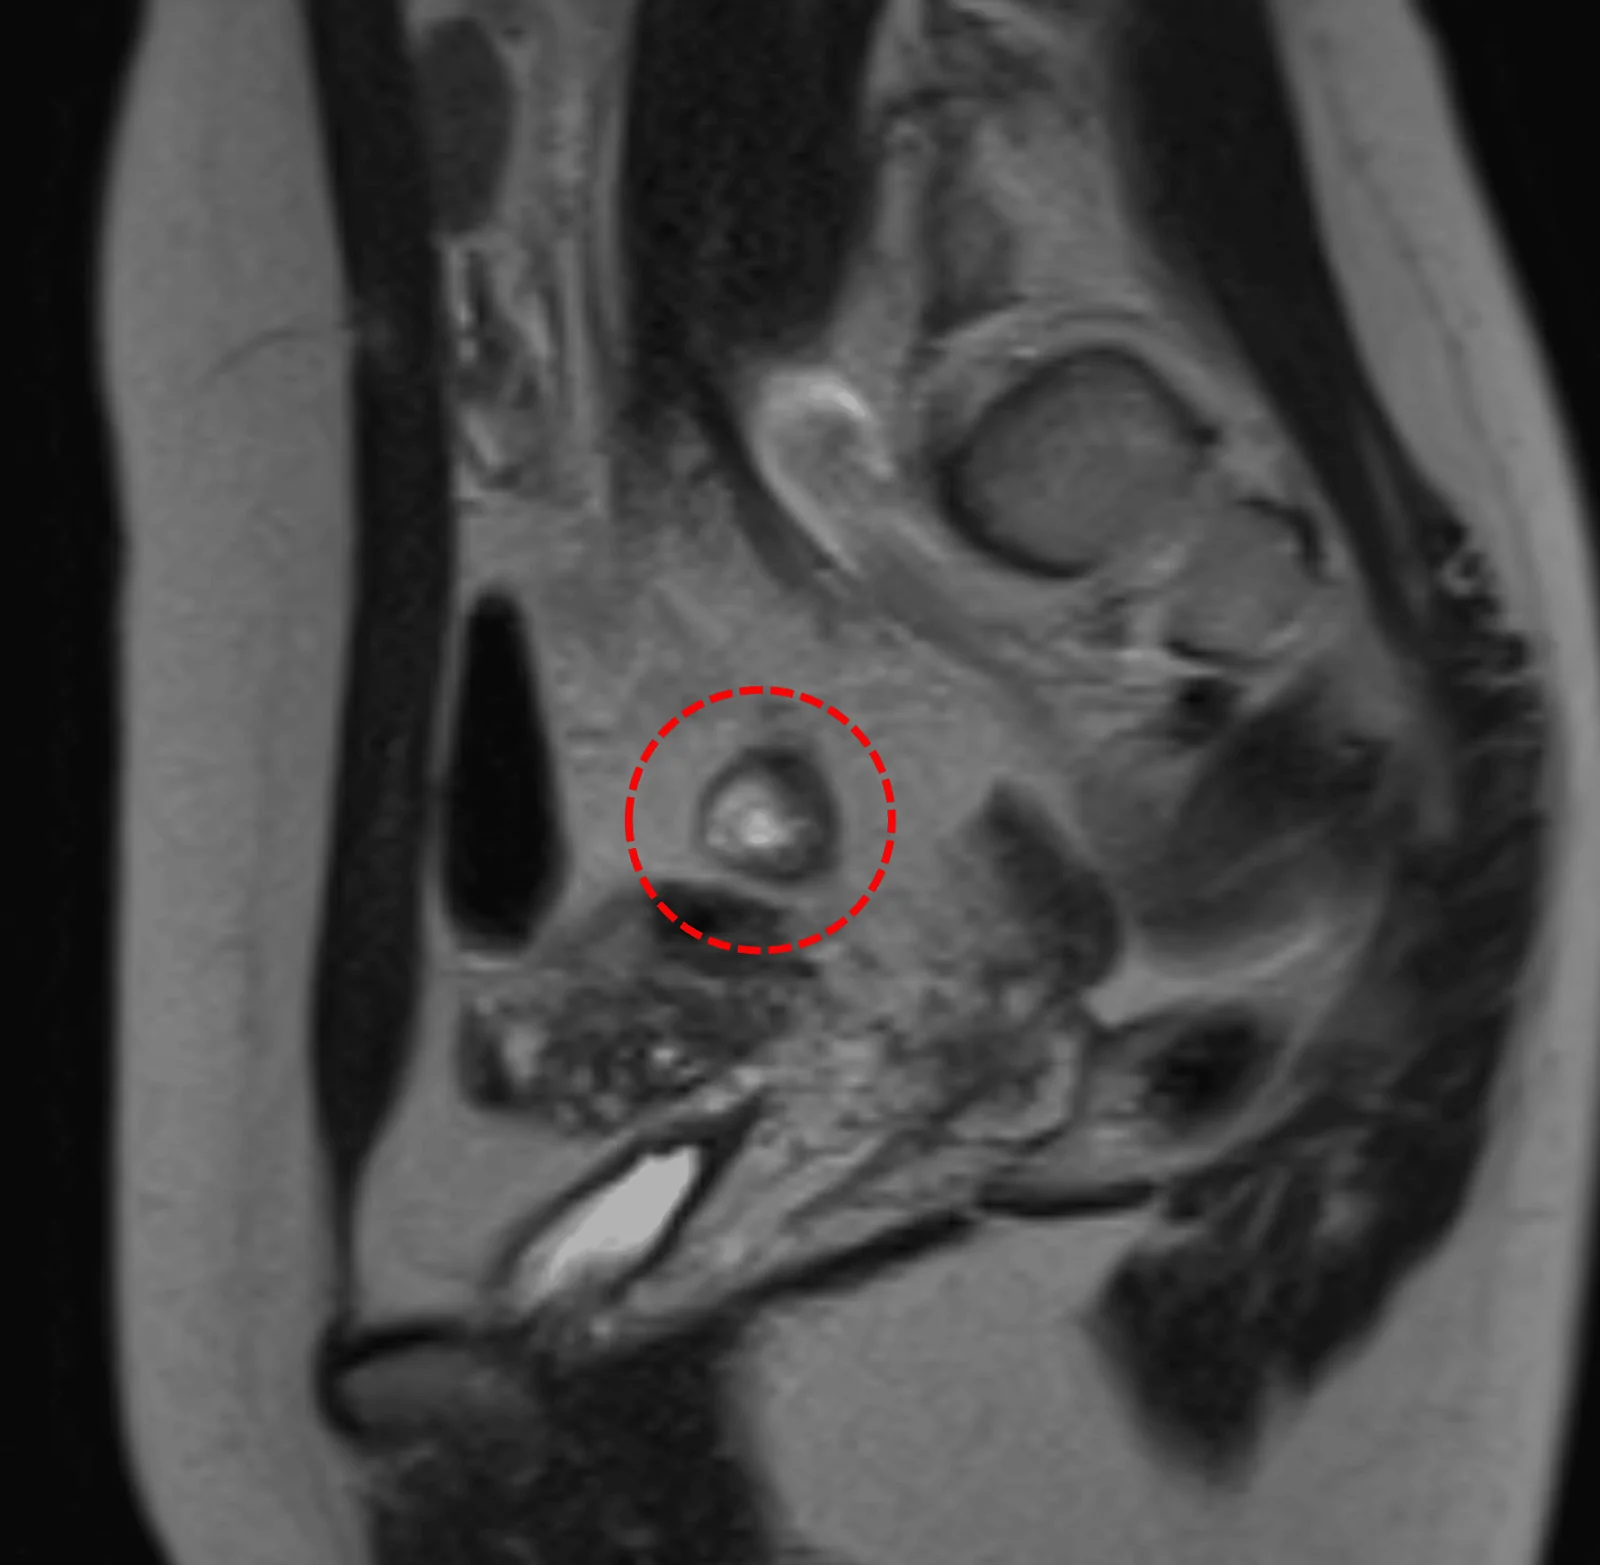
Case 1: Preoperative MRI Preoperative sagittal T2-weighted MRI of Case 1, showing a gestational sac (red dashed circle) in the pelvic cavity, separate from the uterus and adnexa, suggestive of an abdominal ectopic pregnancy.
